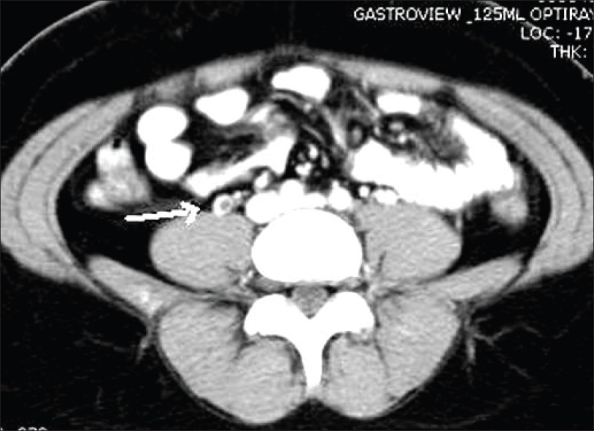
Ovarian vein thrombosis. Contrast-enhanced CT scan shows a filling defect in the right ovarian vein (arrow)
